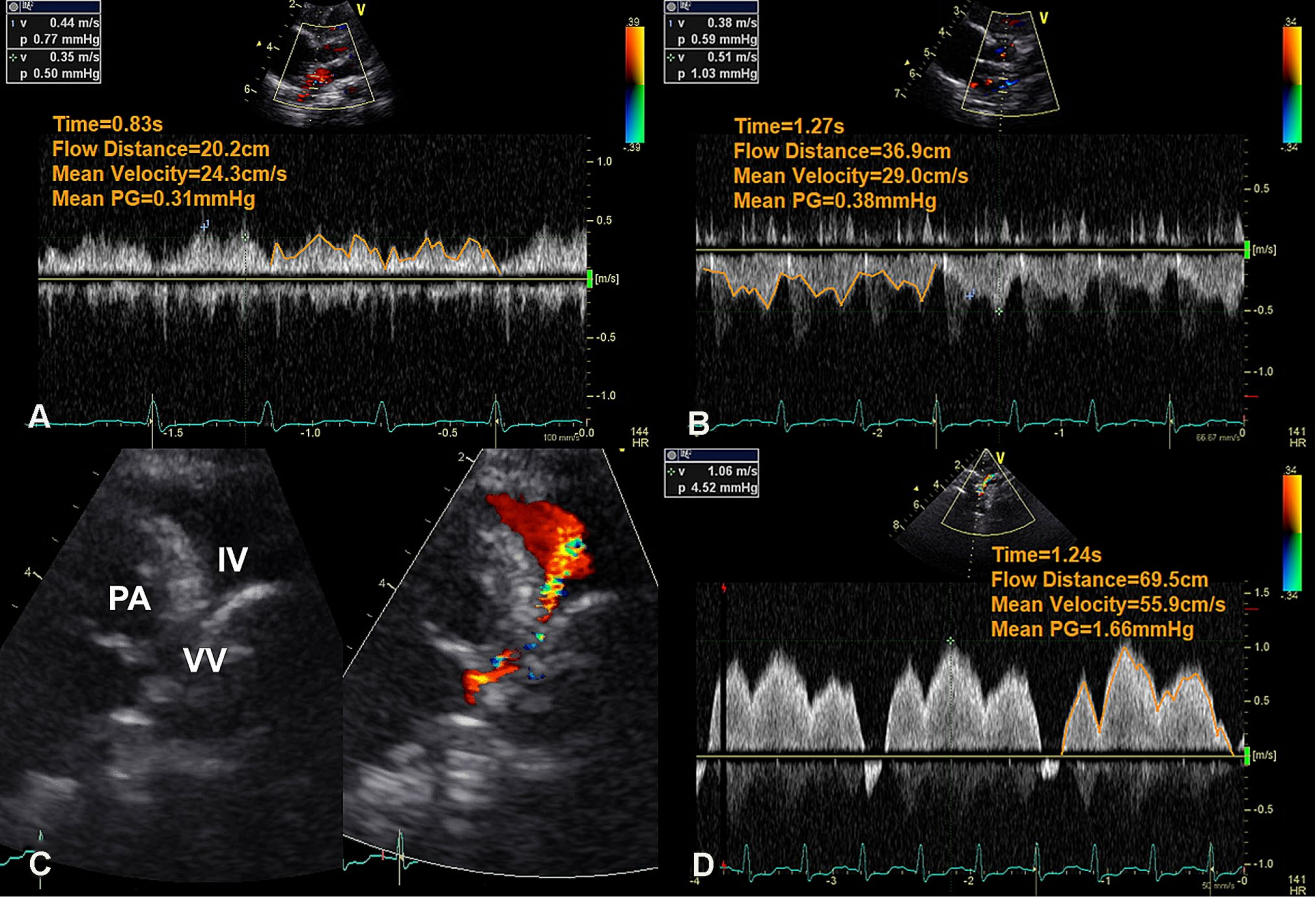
Postoperative transthoracic echocardiography. a Pulse Doppler of phasic flow between the two left atrial chambers. b Pulse Doppler of phasic flow in the common pulmonary venous collector. c The vertical vein (VV) and the turbulent flow in the banding site of the vertical vein as shown color Doppler. d Pulse Doppler of the phasic flow in the banding site of the vertical vein, varying with the cardiac cycle. *IV* innominate vein, *PA* pulmonary artery
